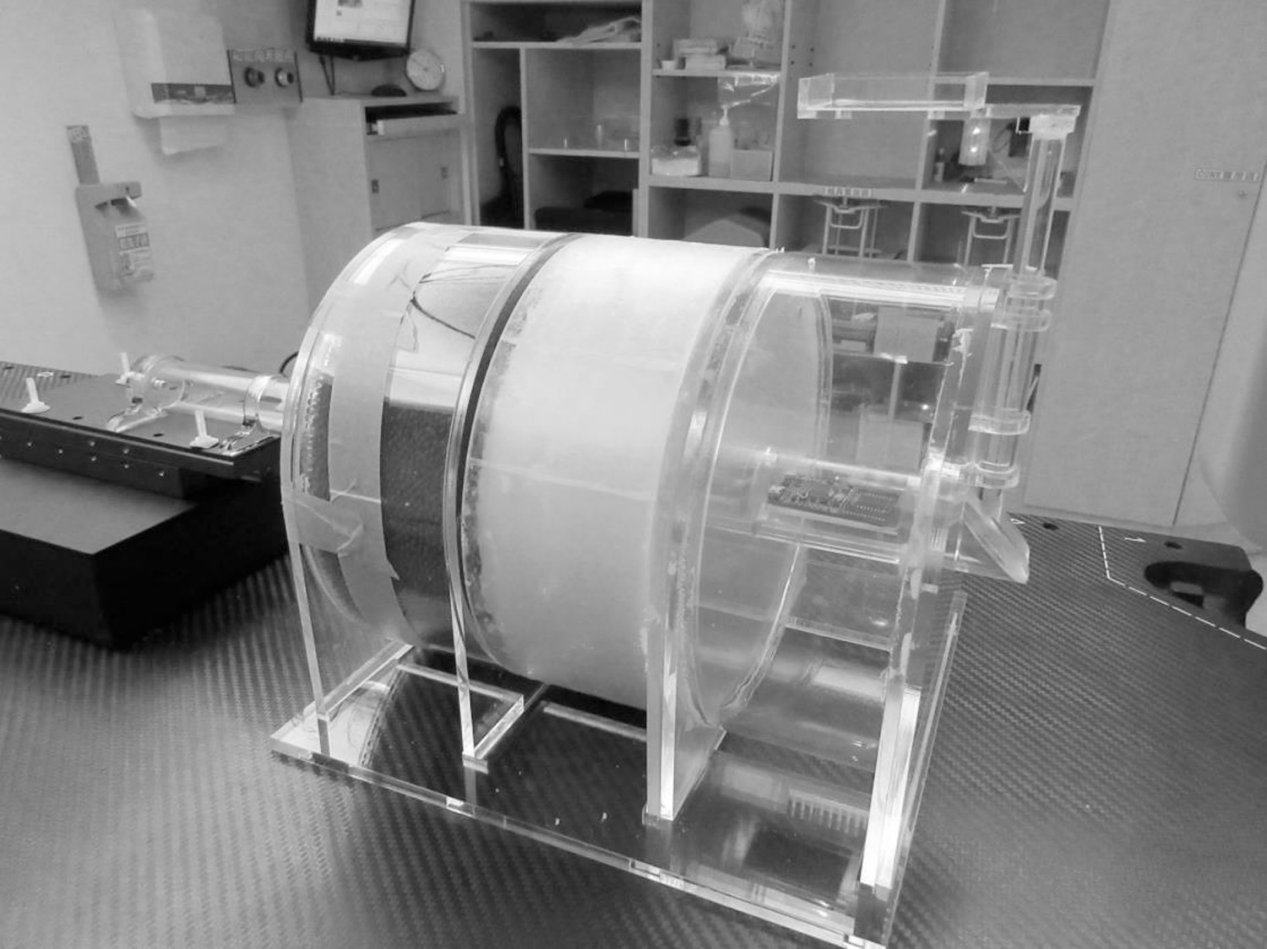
Magnetic resonance imaging module connected to a piezoelectric motor.
